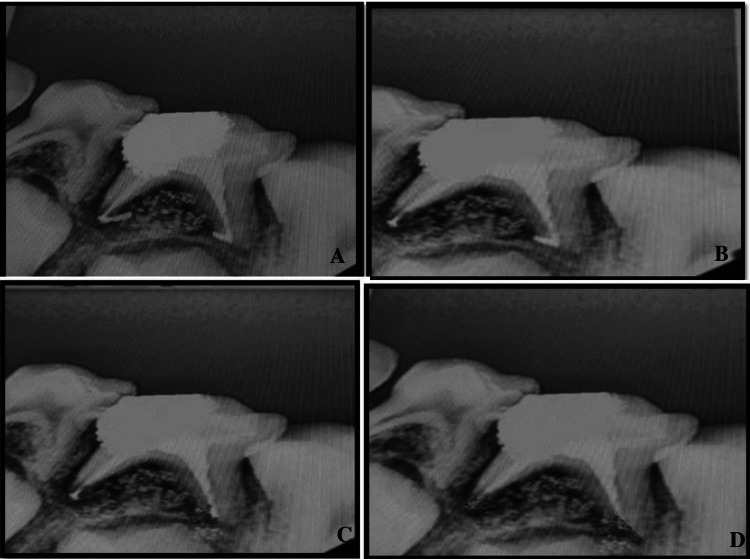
Radiographic evaluation of zinc oxide mixture (A) Immediate postoperative radiograph showing overfilling at root apex. (B) Postoperative radiograph at three months. (C) Postoperative radiograph at six months showing complete resorption of filling material. (D) Postoperative radiograph at nine months.
